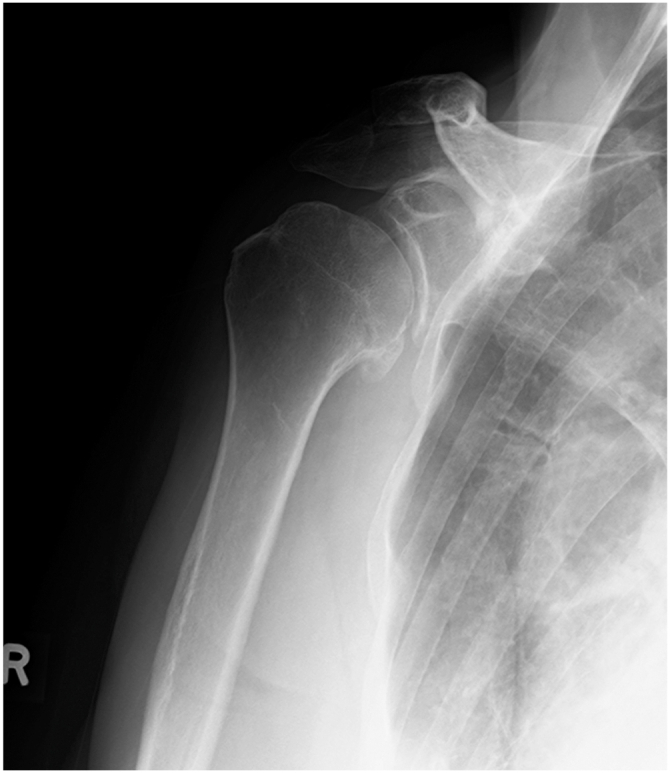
X-ray of a 64-year-old female 14 years after surgery. This X-ray showed moderate osteoarthritic changes.
